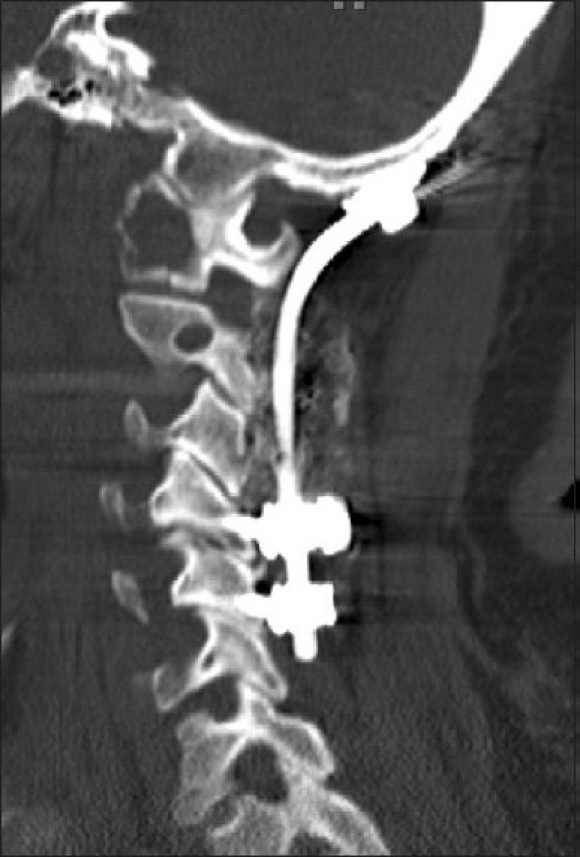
A postoperative sagittal cervical spine CT shows the placement of occiput to C5 instrumentation. (Note that the metastatic NSCLC lesion at the C0-C1 joint was not resected during surgery and that the subsequent alleviation of pain in the patient was achieved through mechanical stabilization of the occipitocervical joint alone)
